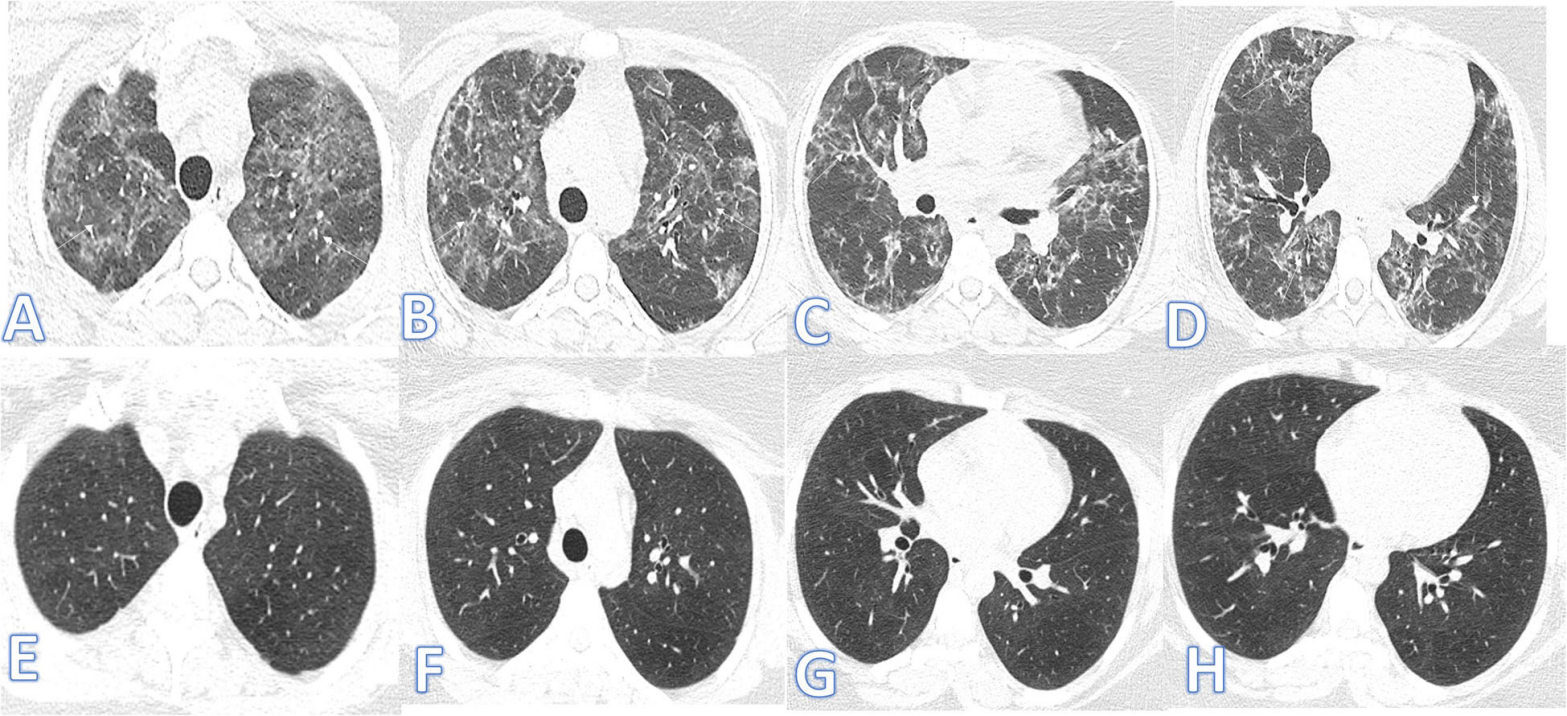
Thirty-year-old female: she has no previous medical illness; CT severity score 15; consolidation/crazy-paving score 10. No ICU admission; laboratory results showed normal lymphocyte and serum ferritin, high CRP, d-dimer. Steroid was given; length of stay during hospitalization is 14 days. Peak CT during admission (a–d) showed bilateral central and peripheral GGO mainly in the upper lobes, associated with lung fibrosis with parenchymal bands and coarse reticular pattern (arrows). Follow-up CT 2 months after discharge (e–h) showed total resolution of the previous lung changes
